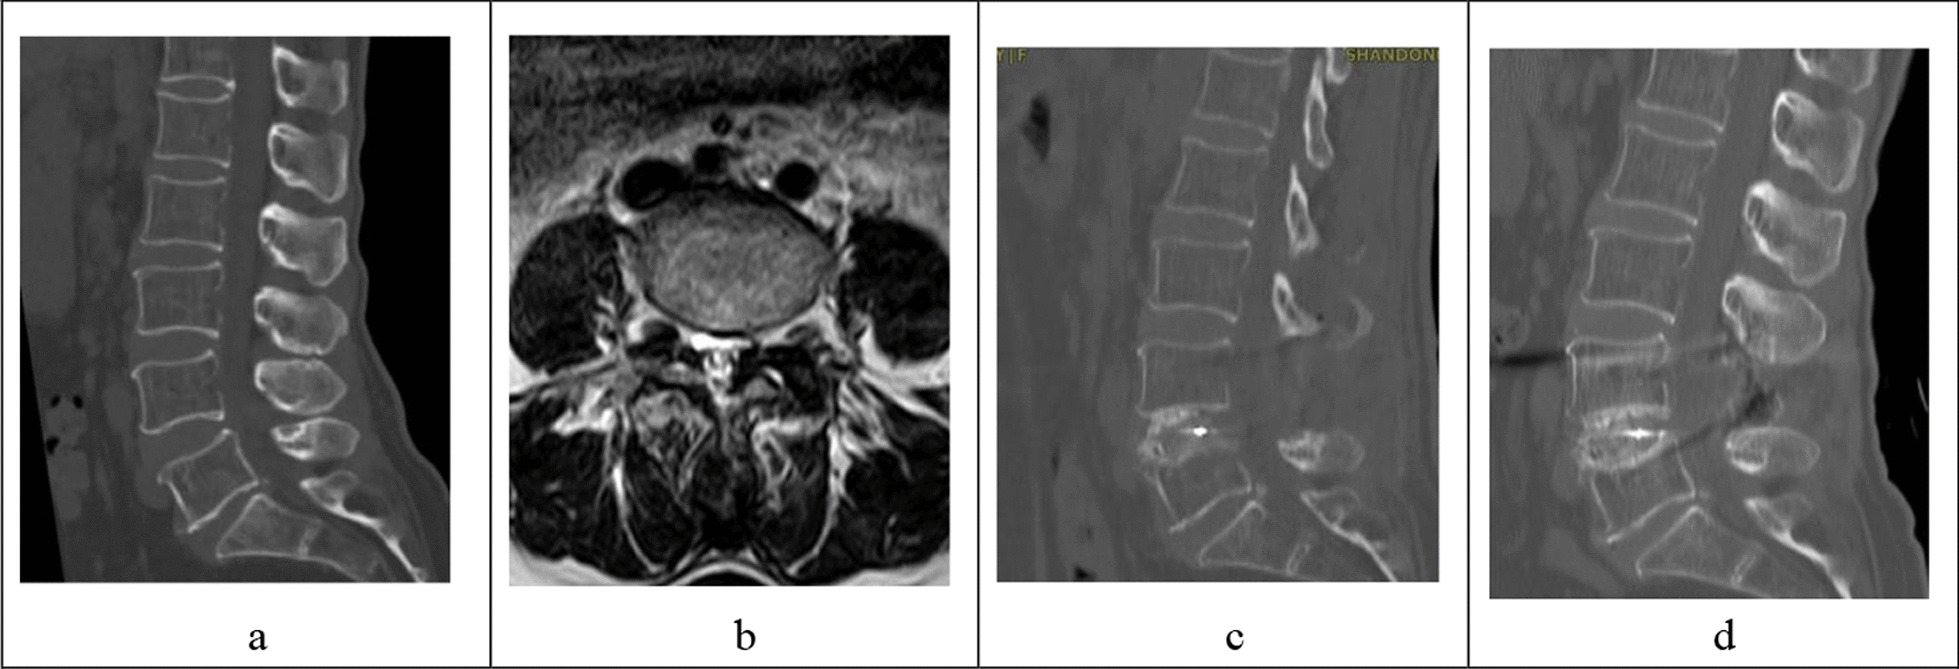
A 74-year-old female patient was admitted to hospital with "lumbar spinal stenosis". Her bone mineral density (BMD) was − 2.6 g/cm^2^. She underwent PLIF surgery and the fusion sink was 3.14 mm after surgery. a: Preoperative CT of the lumbar spine showed that the SL of L4/5 was 12.85°, and the upper and lower endplates were intact. b: Preoperative end plate horizontal MRI under L4 showed that the rCSA of the psoas major muscle was 1.29, the rCSA of the paravertebral muscle was 3.31, the rFCSA of the paravertebral muscle was 2.28, and the vertebral area was 1254.19 mm^2^. c: 1 day after surgery, sagittal CT of lumbar spine showed that the height of intervertebral space correction was 4.01 mm, the Angle of SL correction was 3.61°, and the PI-LL was 3.74°. d: 1 year after surgery, sagittal CT of the lumbar spine showed good intervertebral fusion and significant fusion sink
